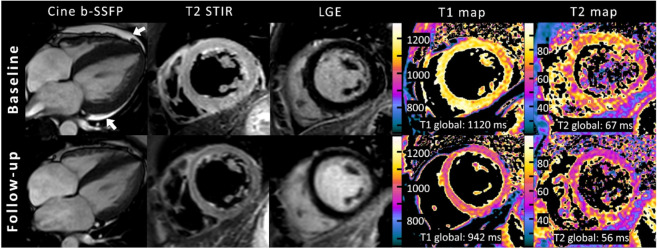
Representative example of cardiac magnetic resonance in a 32-year-old female with acute peripartum cardiomyopathy and recovery at follow-up after 2 months. Cine images (balanced steady-state free precession, b-SSFP) are oriented in horizontal long-axis view and at end systole and showed highly reduced left ventricular ejection fraction (35%) with global hypokinesia, left ventricular dilatation (left ventricular end-diastolic volume index: 118 mL/m²), and pericardial effusion (white arrows). Baseline fat-suppressed images (T2-weighted short TI inversion recovery, T2-STIR) at end diastole revealed extensive diffuse myocardial edema, which normalized at follow-up. No focal enhancement was identified on initial or follow-up late gadolinium enhancement (LGE) imaging. Quantitative mapping showed high global myocardial native T1 and T2 relaxation times at baseline MRI and normalization at follow-up.
